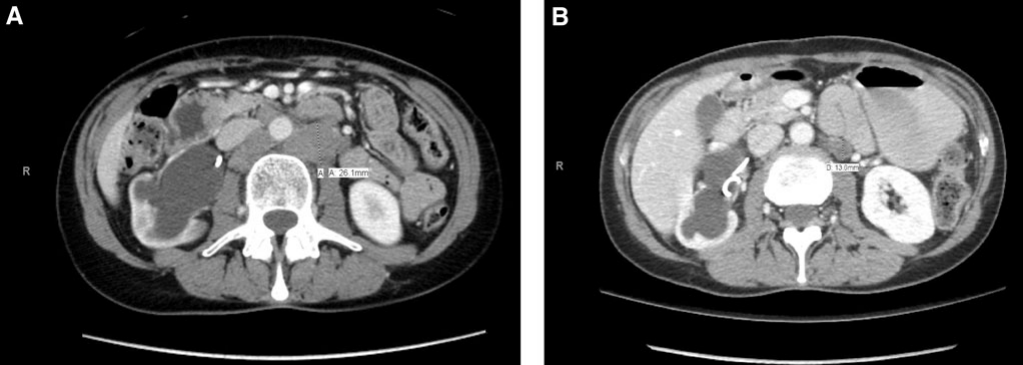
Metastatic work-up after the diagnosis of small cell carcinoma with contrast-enhanced CT of the abdomen–pelvis showing extensive retroperitoneal adenopathy (A), response to chemotherapy demonstrating ∼50% reduction in the lymph nodal burden (B).
